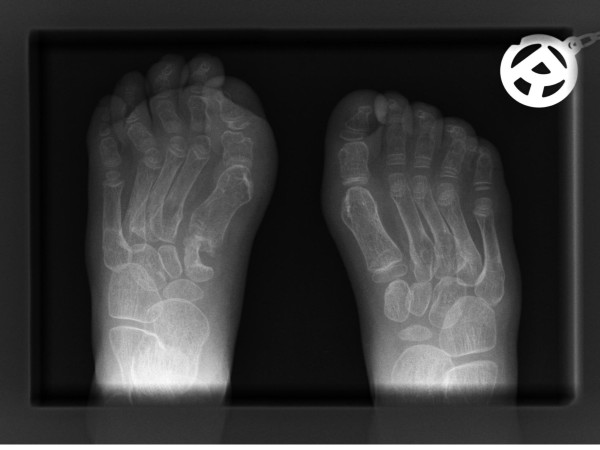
Anteroposterior radiograph of the foot showed widespread erosions and shortenings of the big toes associated with osteolysis of the tarsals and erosions of the metatarsals and the cortices were thin.
